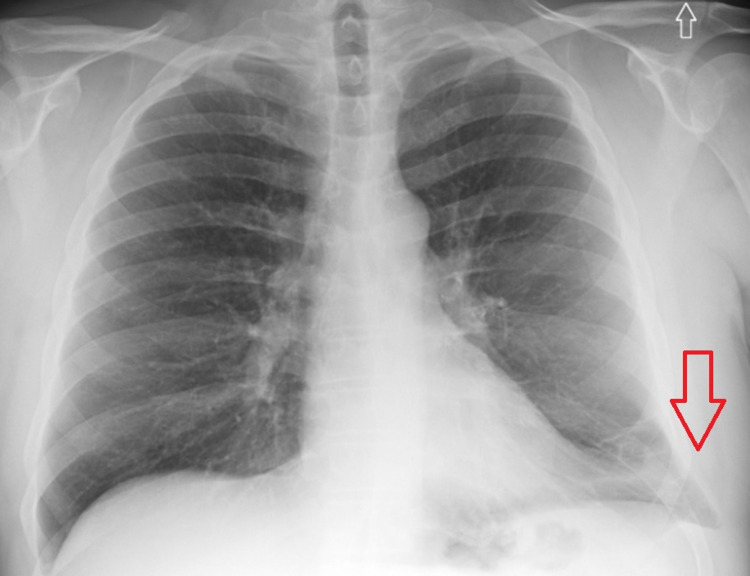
Posteroanterior chest radiography evidencing protrusion of pulmonary tissue beyond costal margins in the left lower lung aspect (red arrow).
